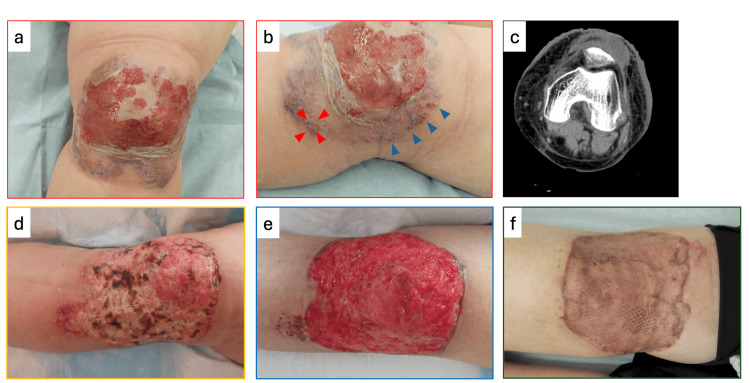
Clinical photographs _(a, b) Initial presentation at our hospital, showing an erosive ulcer with vesicle formation and knee swelling. Pustules were observed at the lesion periphery (red and blue arrowheads)._ _(c) Computed tomography at initial presentation revealed hyperintensity in the subcutaneous fat tissue without evidence of fluid collection._ _(d) Post-surgical debridement; fever and laboratory data remained unchanged. Oral prednisolone (60 mg/day) was initiated on postoperative day 4 (POD4), with subsequent rapid improvement in symptoms and markers._ _(e) Skin ulcer flattened following negative pressure wound therapy (RENASYS®; Smith & Nephew plc, London, United Kingdom)._ _(f) Successful split-thickness skin graft performed without complications. Adalimumab (ADA) 160 mg was initiated to prevent relapse and facilitate prednisolone tapering._
